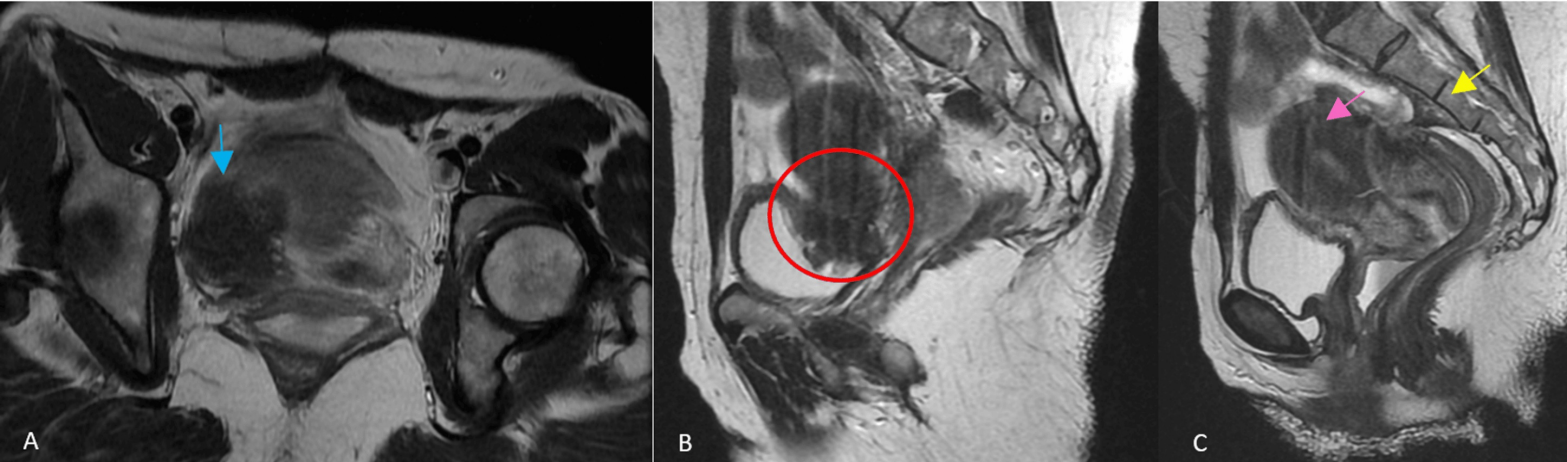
Pelvic T2-weighted MRI images, including an axial view (A) and sagittal views (B and C), demonstrating imaging findings consistent with deep infiltrating endometriosis. The lesions appear as nodular and plaque-like areas of low signal intensity on T2-weighted images, reflecting fibrotic endometriotic implants. Endometriotic lesions involve the urinary bladder (blue arrows) and the vesicouterine pouch (red circle) and extend to the level of the uterine torus (yellow arrow), with associated retraction of adjacent pelvic structures. Additional MRI features suggestive of adenomyosis are also observed, including thickening of the uterine junctional zone (pink arrow).
